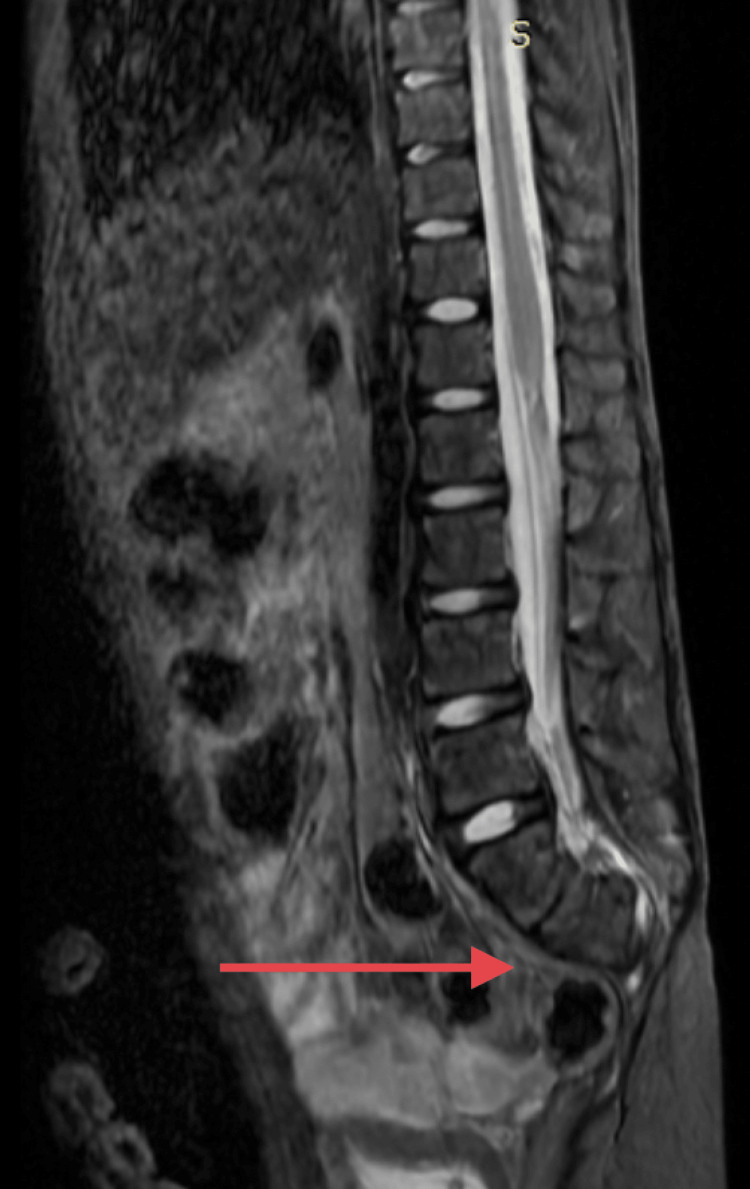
MRI spine sagittal section showing absent sacral vertebrae below the level of the second sacral vertebra MRI: magnetic resonance imaging
